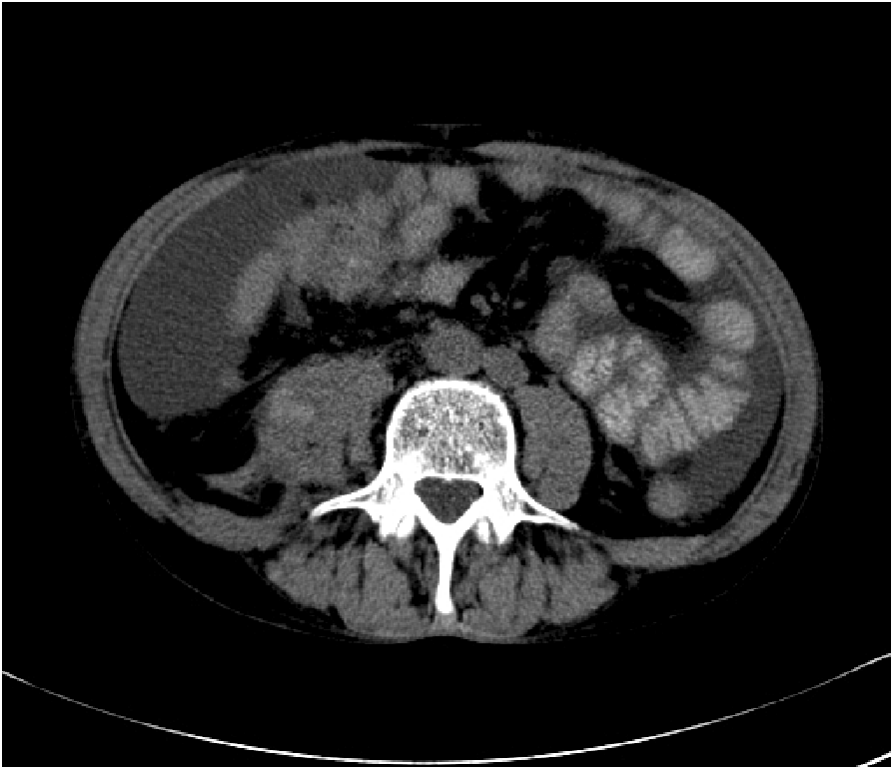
MM type V in a patient with known history of esophageal cancer. CT without intravenous administration of contrast medium shows hyperdense areas (arrow) in the right iliopsoas muscle.
